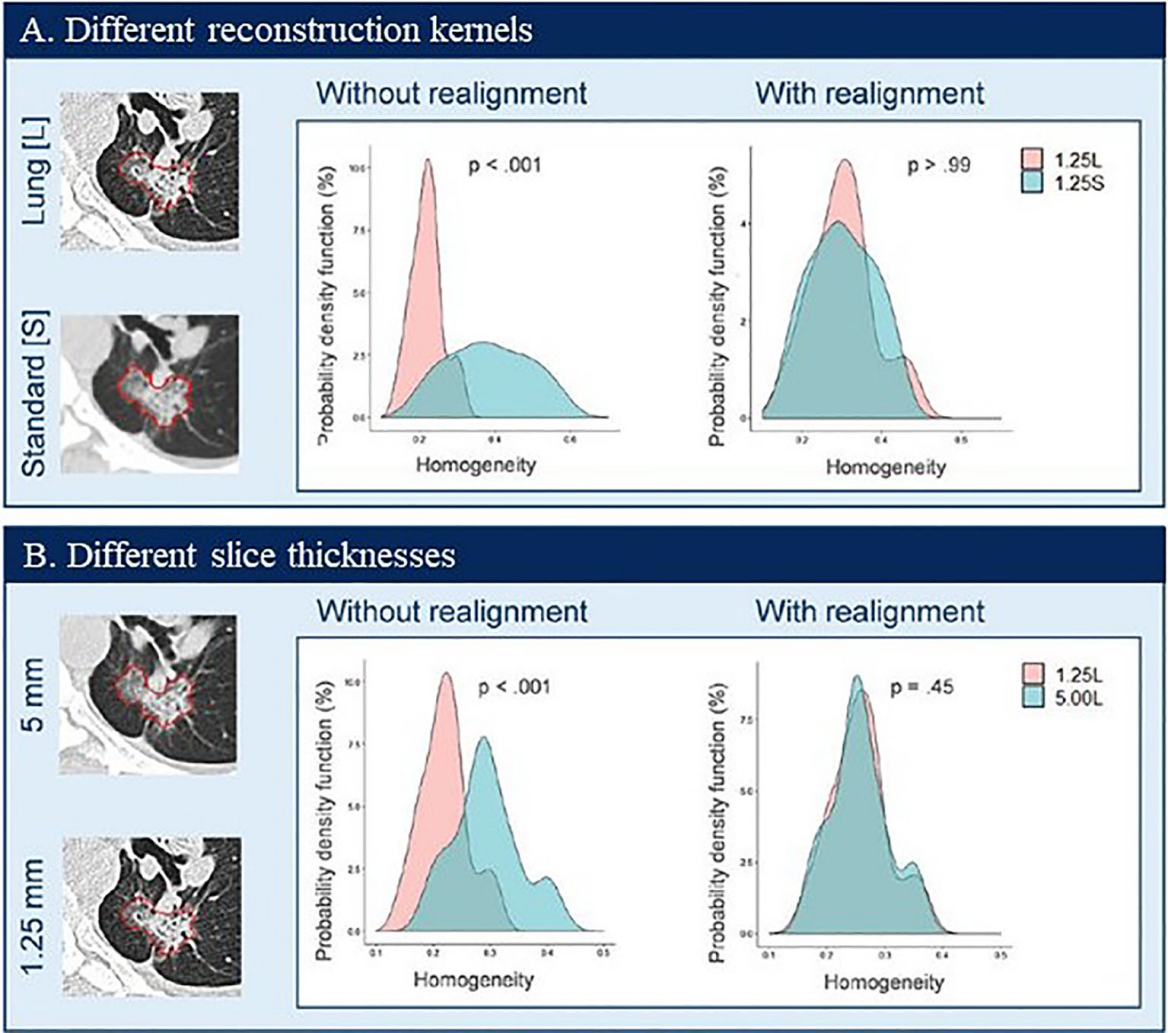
Probability density distributions of Homogeneity before (without realignment) and after (with realignment) ComBat in patient data by using two CT reconstruction kernels (A) and two slice thicknesses (B). Reproduced with permission from (108).
